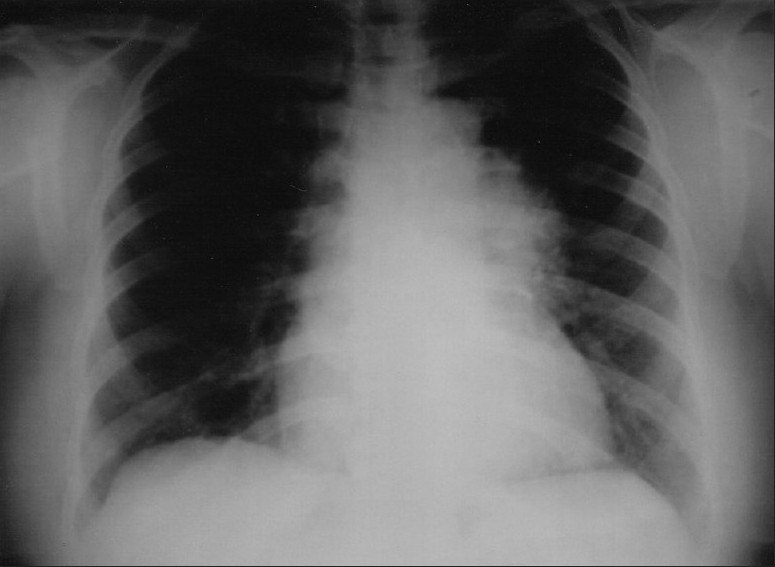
X-ray chest, PA view, showing non-homogenous mass lesion near the left hilum
